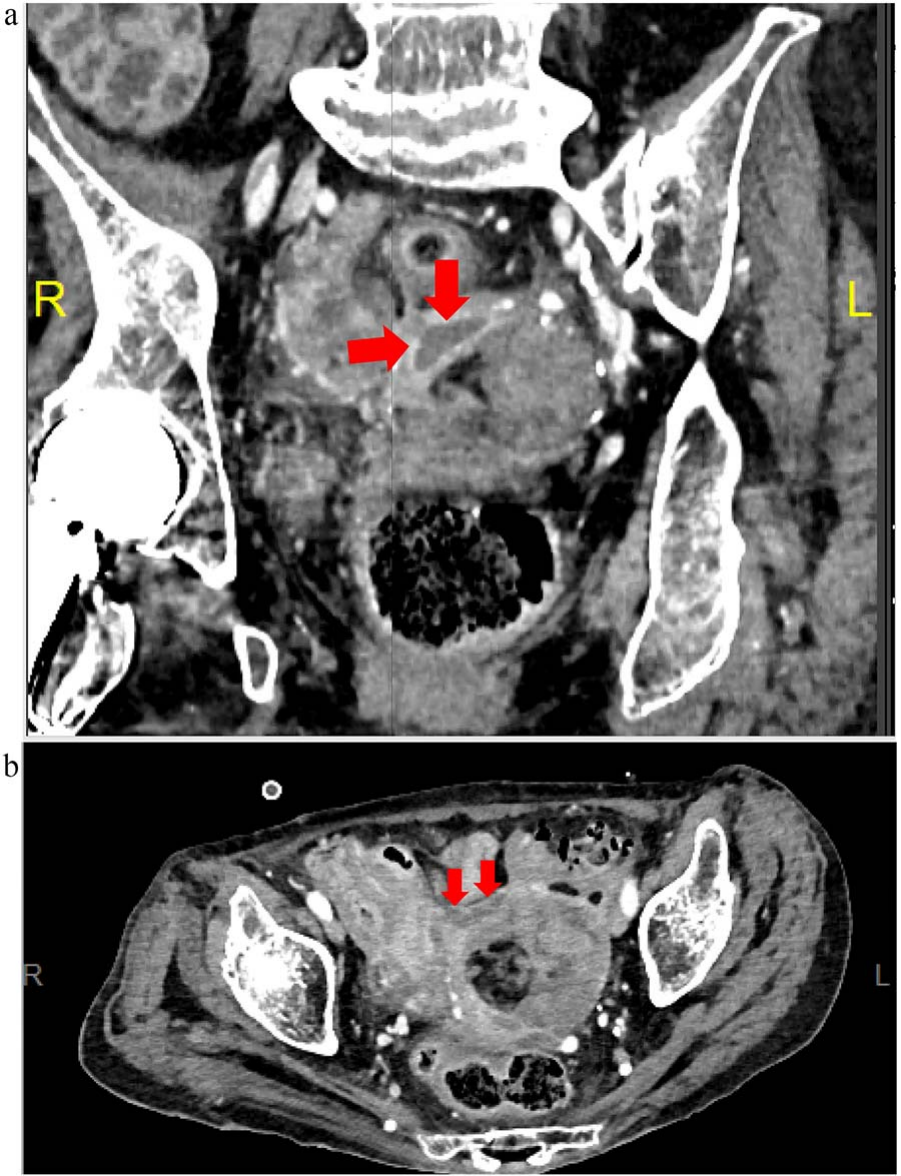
Postoperative Day 18 contrast-enhanced CT images (equilibrium phase). (a) Coronal view; (b) horizontal view.
